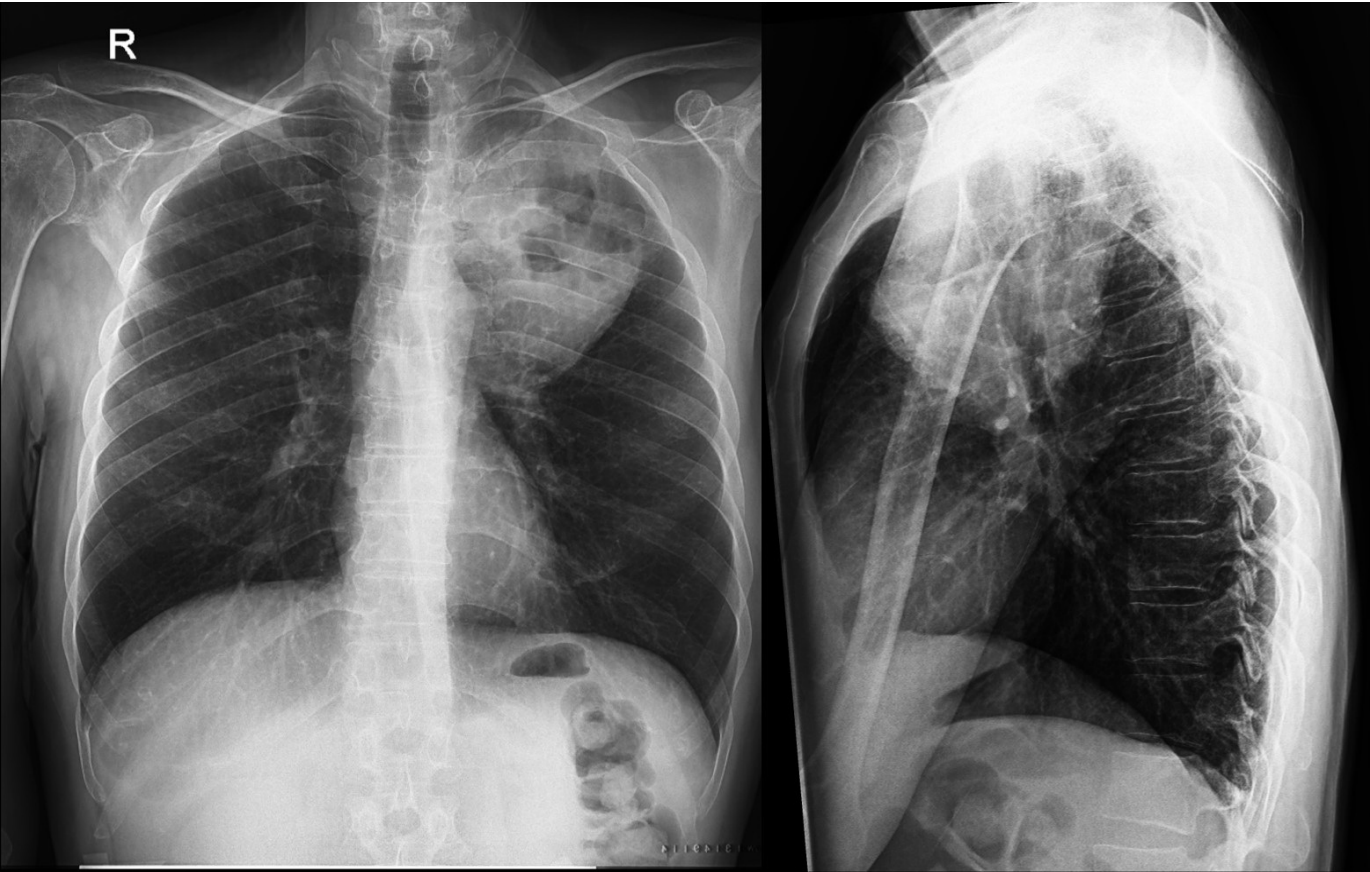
Brain CT scan showing the brain resected lesion in the left parietal lobe
